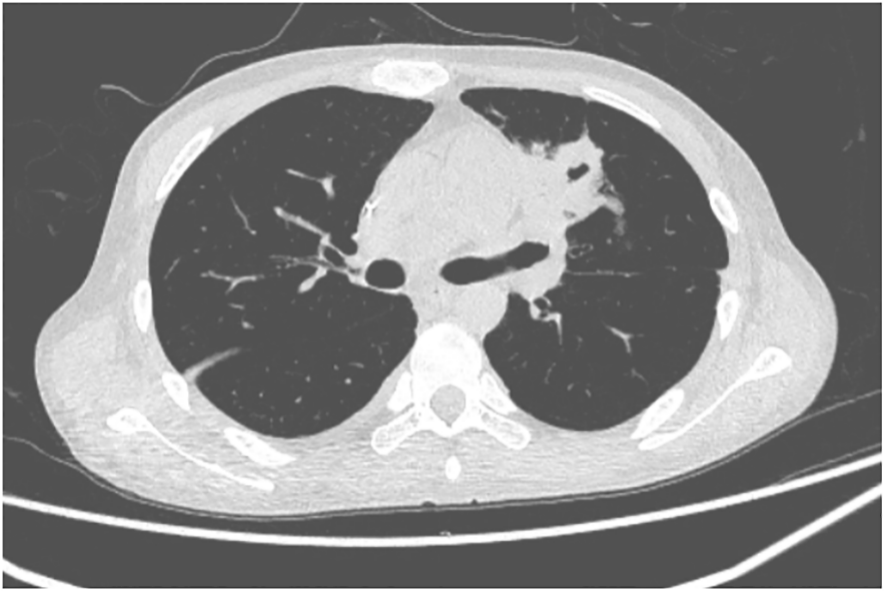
Case 2 lung CT on day +48.
